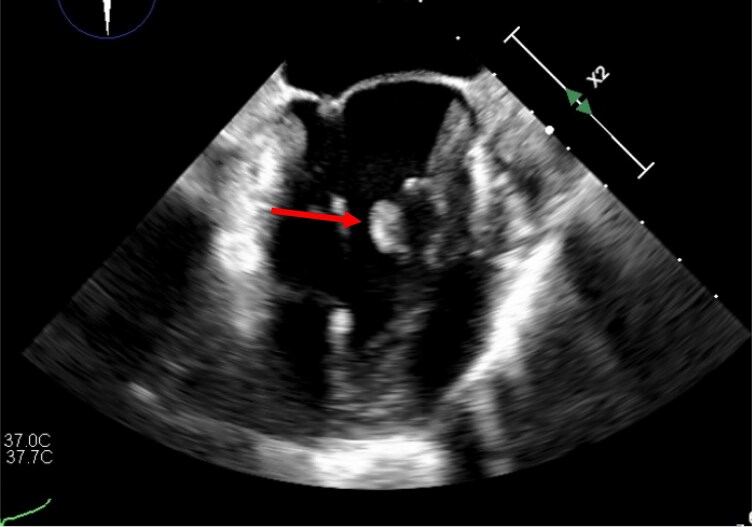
Transoesophageal echocardiography showing a mobile intracardiac mass on the posterior papillary muscle (arrow).
